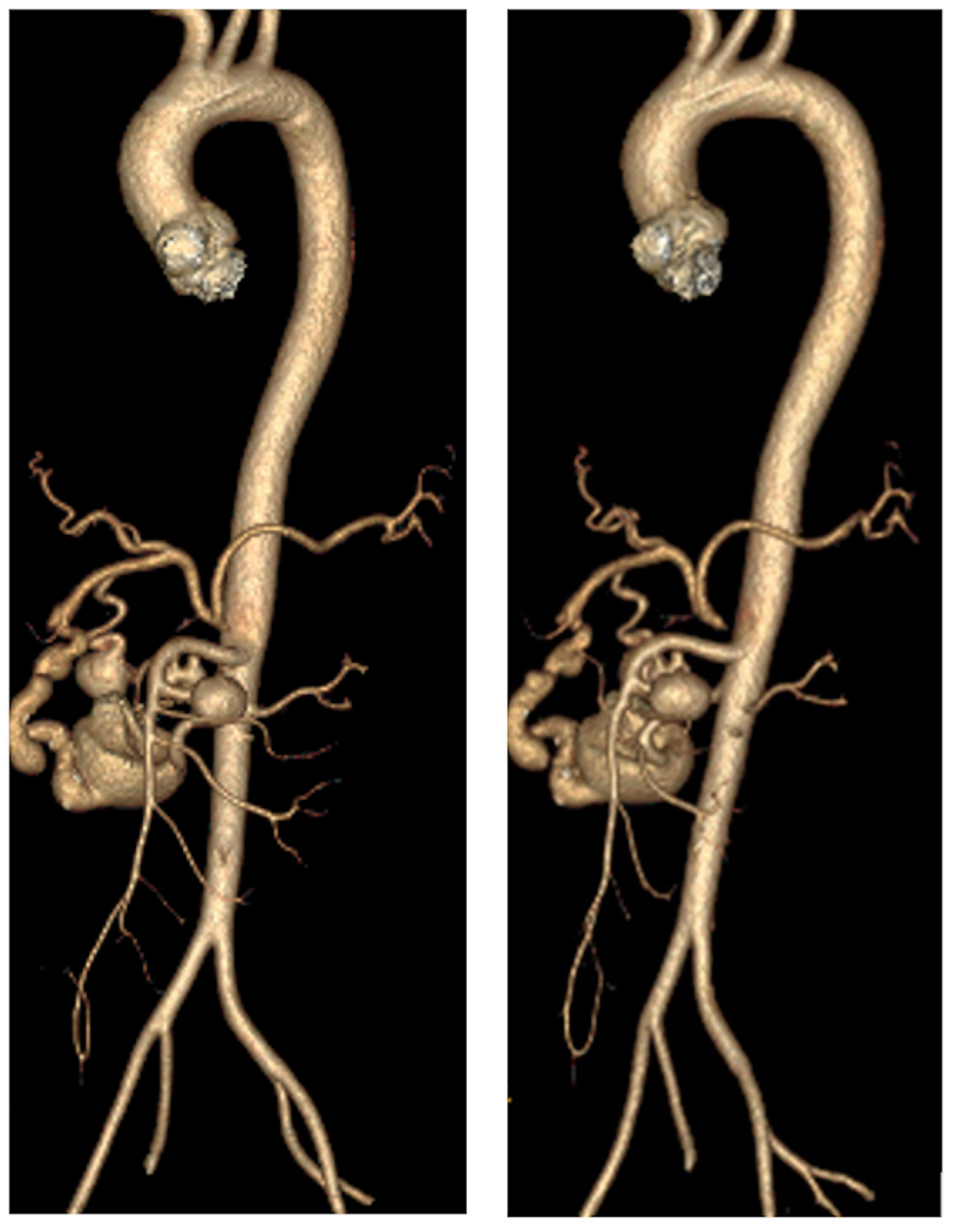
Three-dimensional reconstruction of computed tomography (CT) angiography demonstrating one aneurysm of the gastroduodenal artery (4.6 cm), two of the superior mesenteric artery (1.7 cm, <1.0 cm), and two of pancreaticoduodenal arcades (1.3 cm, 1.5 cm) with concurrent celiac occlusion.
